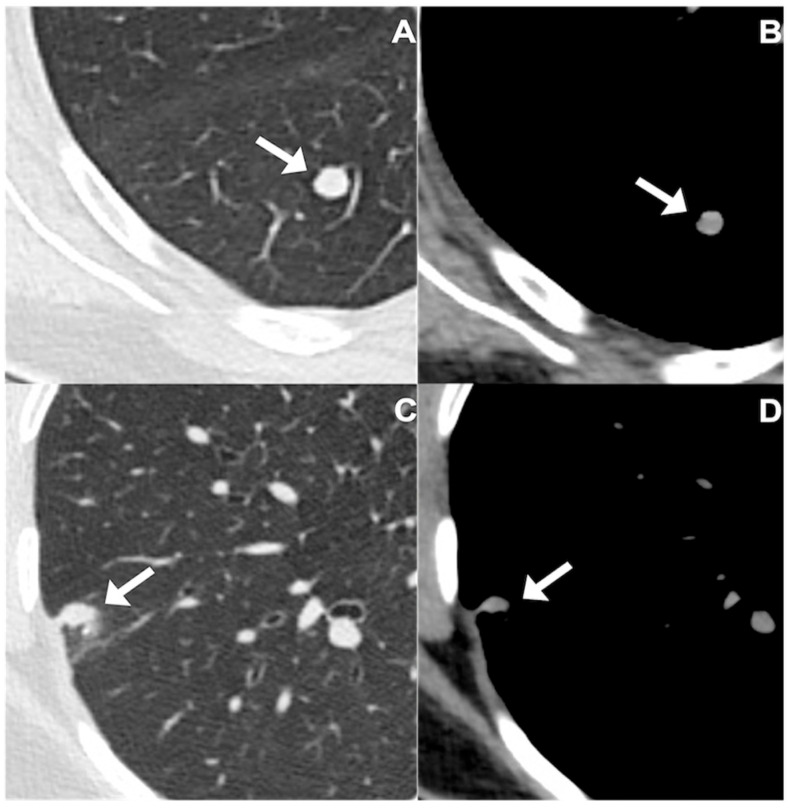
(A) Lung and (B) mediastinal window settings on a non-contrast chest CT in a 54-year-old male. The nodule (arrow) shows round shape, regular margins, no spiculation. After surgery, a pathological diagnosis of tumorlet was given. (C) Lung and (D) mediastinal window of unenhanced chest CT in 67-years-old female patient. The nodule (arrow) shows ovalar shape, feather edges, regular margins, and no spiculation. After surgery, a pathological diagnosis of tumorlet was given.
